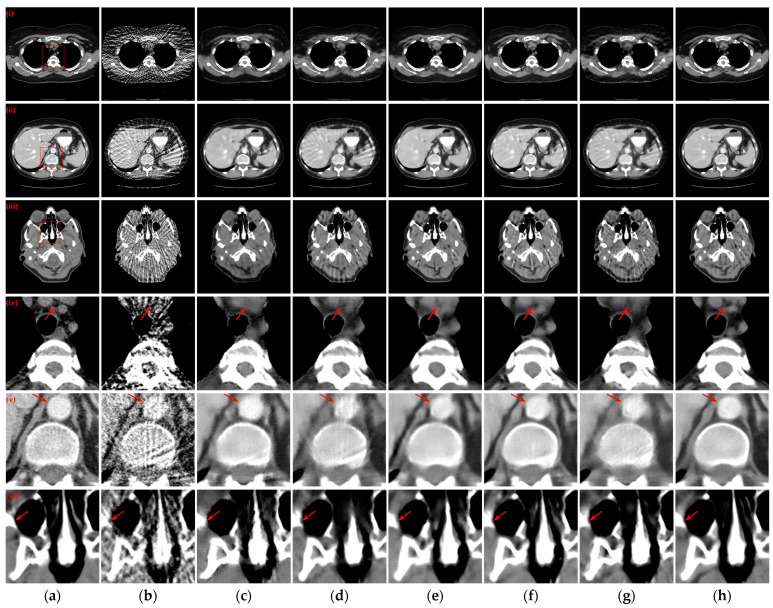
The SVCT reconstruction results from different methods with 64 projection views. (a) The reference image; (b) FBP; (c) MWNet; (d) DuDoTrans; (e) DDPTransformer; (f) MIST; (g) RegFormer; (h) Ours. (i)–(iii) are the chest CT, abdominal CT, and head CT, respectively, and (iv)–(vi) correspond to the enlarged ROIs marked by the red boxes in the reference images above. The display window is [−160, 240] HU.
